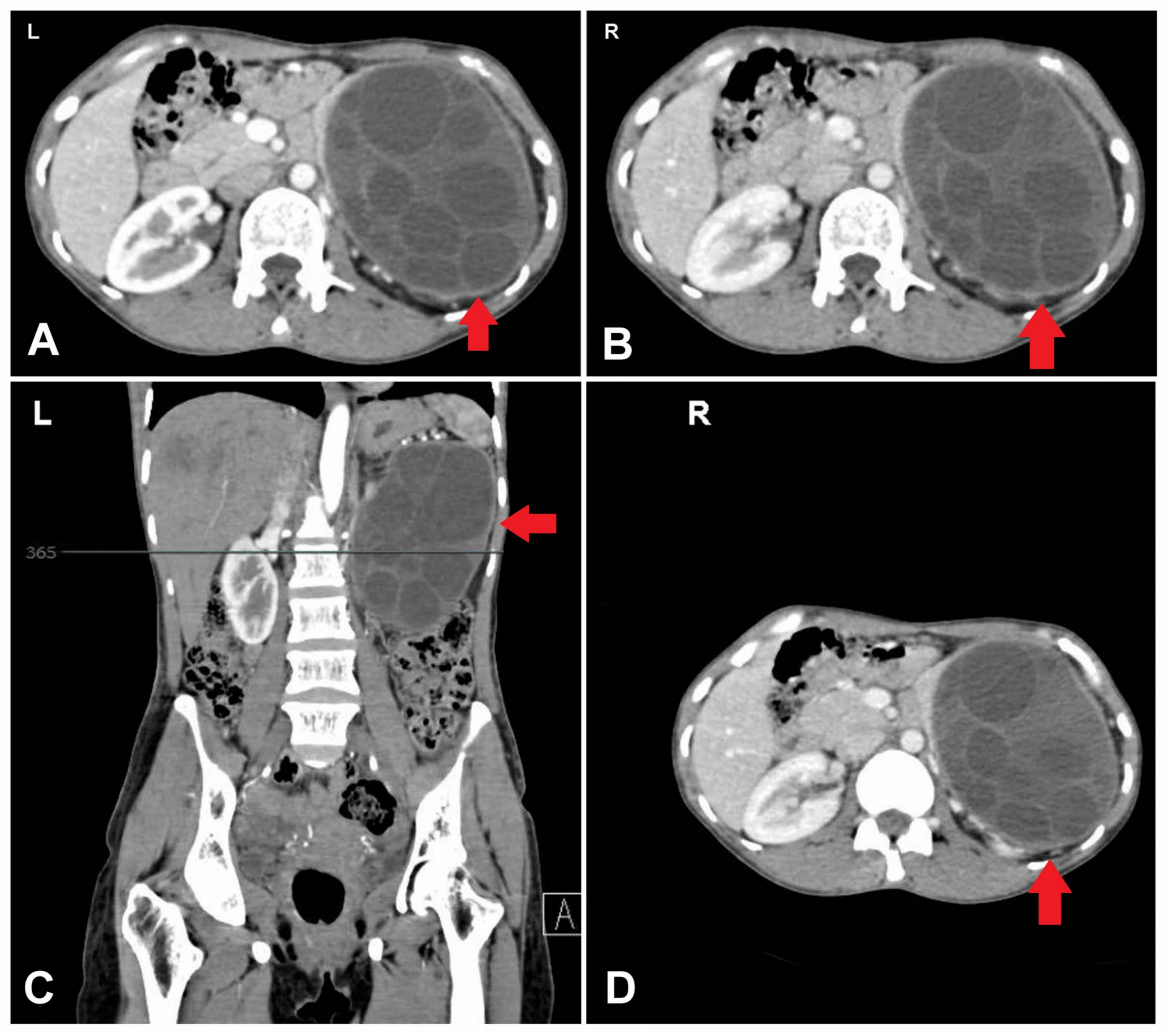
(A-D) CECT abdomen shows cyst measured 9.8 x 10.8 x 13.7 cm and was compressing the left kidney and the tail of the pancreas, closely abutting the splenic and left renal vessels. CECT: Contrast-enhanced computed tomography
